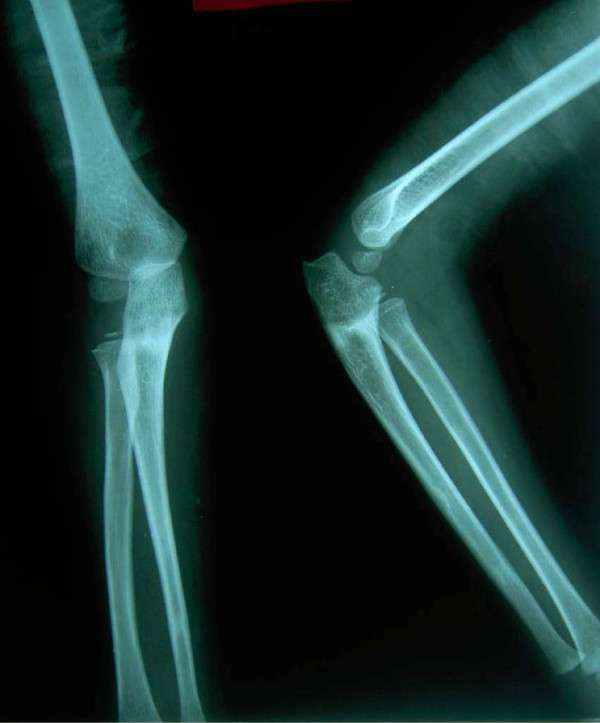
Radiograph taken at the two-year follow-up visit showng excellent relocation of the radial head (Patient 1).
